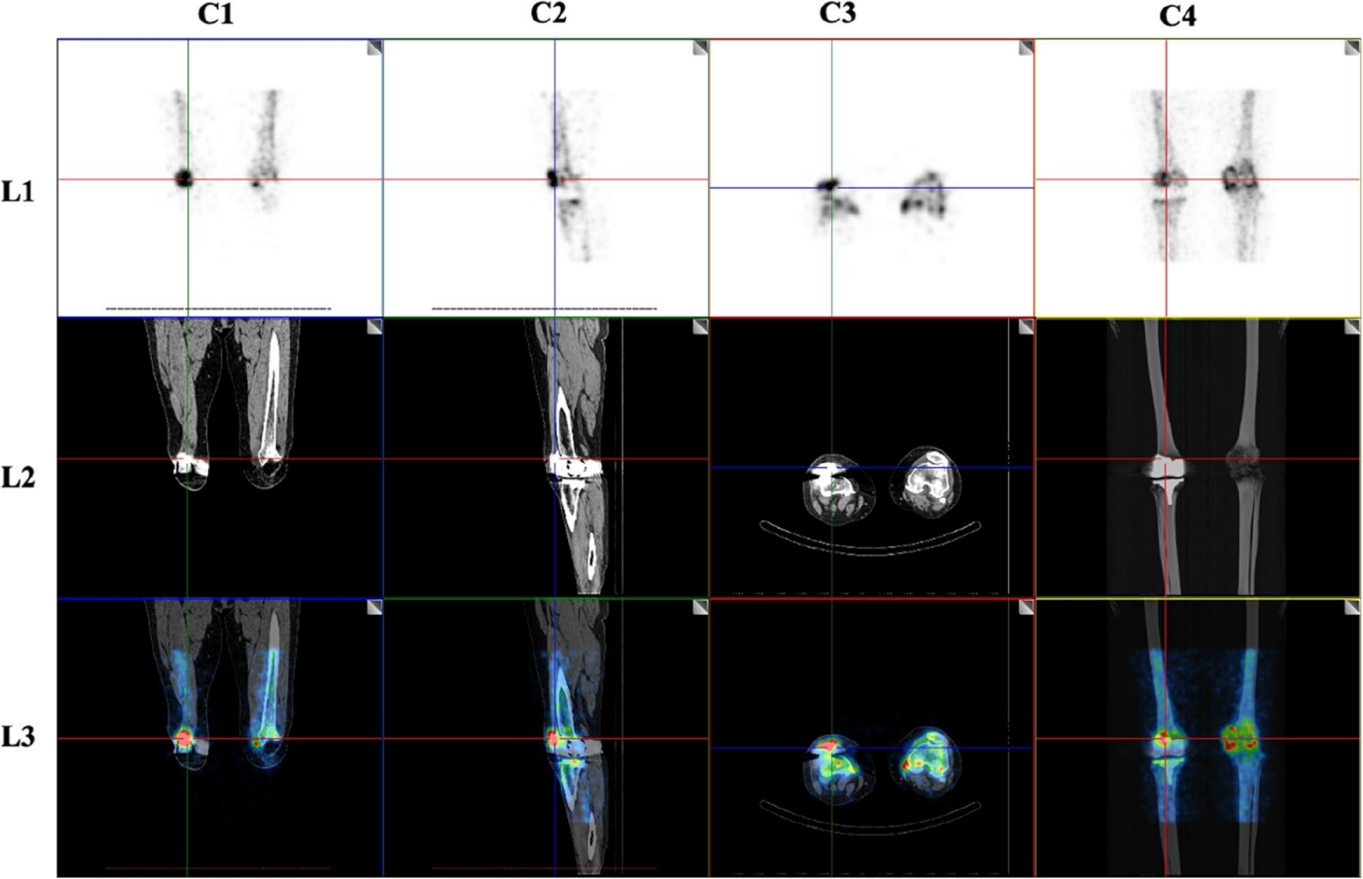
SPECT/CT Imaging of the Knee. The images are presented in various orthogonal planes: coronal (C1), sagittal (C2), and orthogonal axial (C3) planes, alongside a 3D reconstruction (C4). Concurrently, the figure includes images of bone scintigraphy (L1), CT scan (L2), and reconstructed images with a color-coded grading scale (L3)
